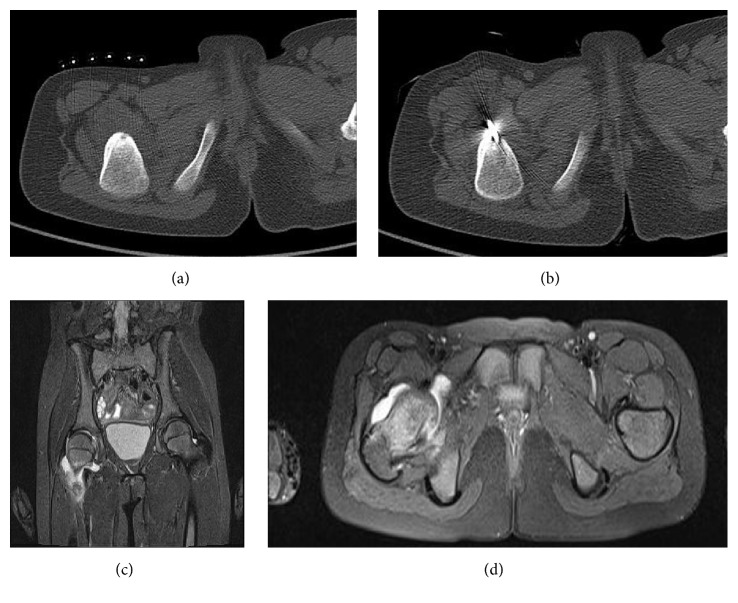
(a)-(b) 8-year old girl patient with CT image of the lesion in right femoral neck. (c)-(d) T2 MR images at 4th week after the procedure.
